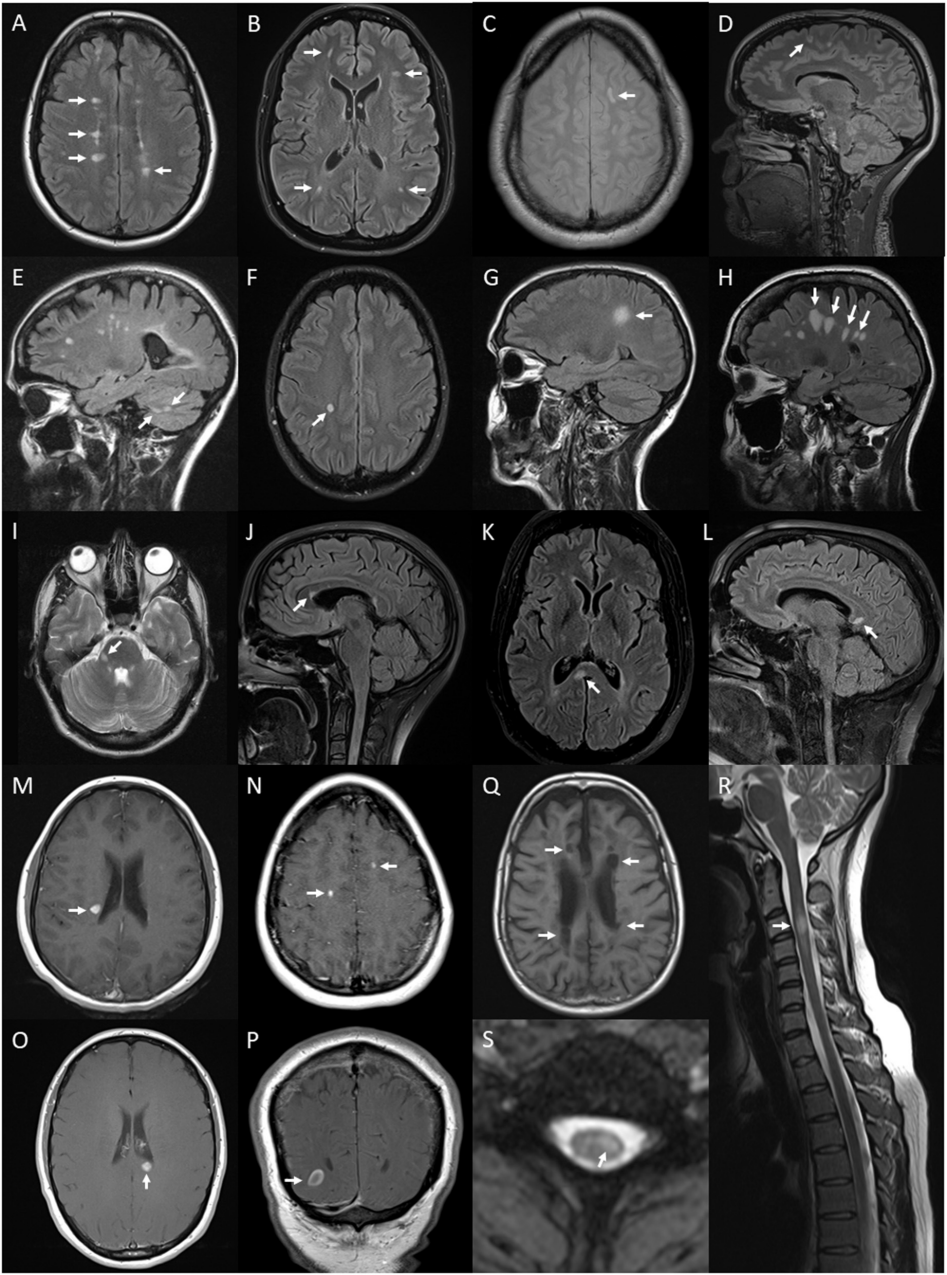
Lesions and features on MRI of brain and spinal cord associated with multiple sclerosis: periventricular hyperintense T2 white matter lesions (arrows) on axial FLAIR image of the brain (A); subcortical T2 white matter lesions (arrows) on axial FLAIR image of the brain (B); juxtacortical hyperintense T2 white matter lesion (arrow) on axial proton density image of the brain (C); cortical hyperintense T2 lesion (arrow) on sagittal FLAIR image of the brain (D); cerebellar hyperintense T2 lesions (arrows) on sagittal FLAIR image of the brain (E); ovoid hyperintense T2 periventricular lesion (arrow) on axial FLAIR image of the brain (F); large supratentorial T2 lesion (arrow) on sagittal FLAIR image of the brain (G); Dawson's finger lesions (arrows) on sagittal FLAIR image of the brain (H); right cerebellar peduncle hyperintense T2 lesion (arrow) on axial T2 image of the brain (I); pyramidal corpus callosum hyperintense T2 lesion (arrow) on sagittal FLAIR image of the brain (J); splenium hyperintense T2 lesion (arrow) on axial (K) and sagittal (L) FLAIR images of the brain; Gd-enhancing T1 lesions of periventricular (arrow) (M), juxtacortical (arrows) (N), splenium (arrow) (O) regions on axial T1 images of the brain and ring-enhancing lesion (arrow) on coronal T1 image of the brain (P); hypointense T1 (black hole) lesions (arrows) on axial T1 image of the brain (Q); short segment C3 T2 lesion (arrow) on sagittal T2 image of the cervical spinal cord (R); and hyperintense T2 partial cord lesion (arrow) on axial T2 image of the cervical spinal cord (S).
